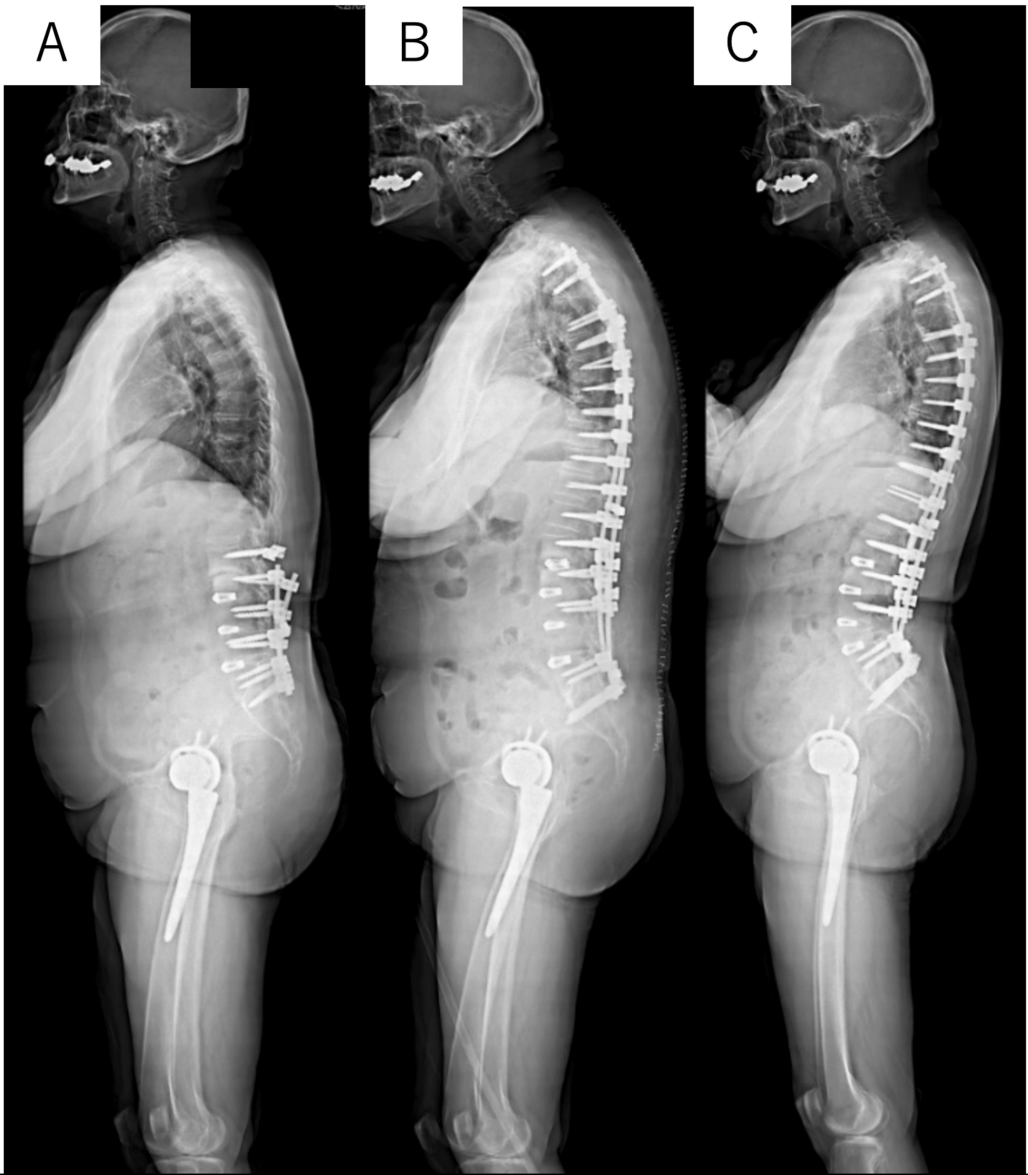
Standing lateral radiograph of the entire spine of a 72-year-old woman. (A) Preoperative standing lateral radiograph of the entire spine. Iatrogenic kyphosis, a fixed lumbar spine, and minimal kyphosis are present. (B) Postoperative (posterior corrective fixation of T2–S2) standing lateral radiograph of the entire spine. (C) Postoperative (pedicle subtraction osteotomy at L5) standing lateral radiograph of the entire spine. Good spinal alignment is observed.
